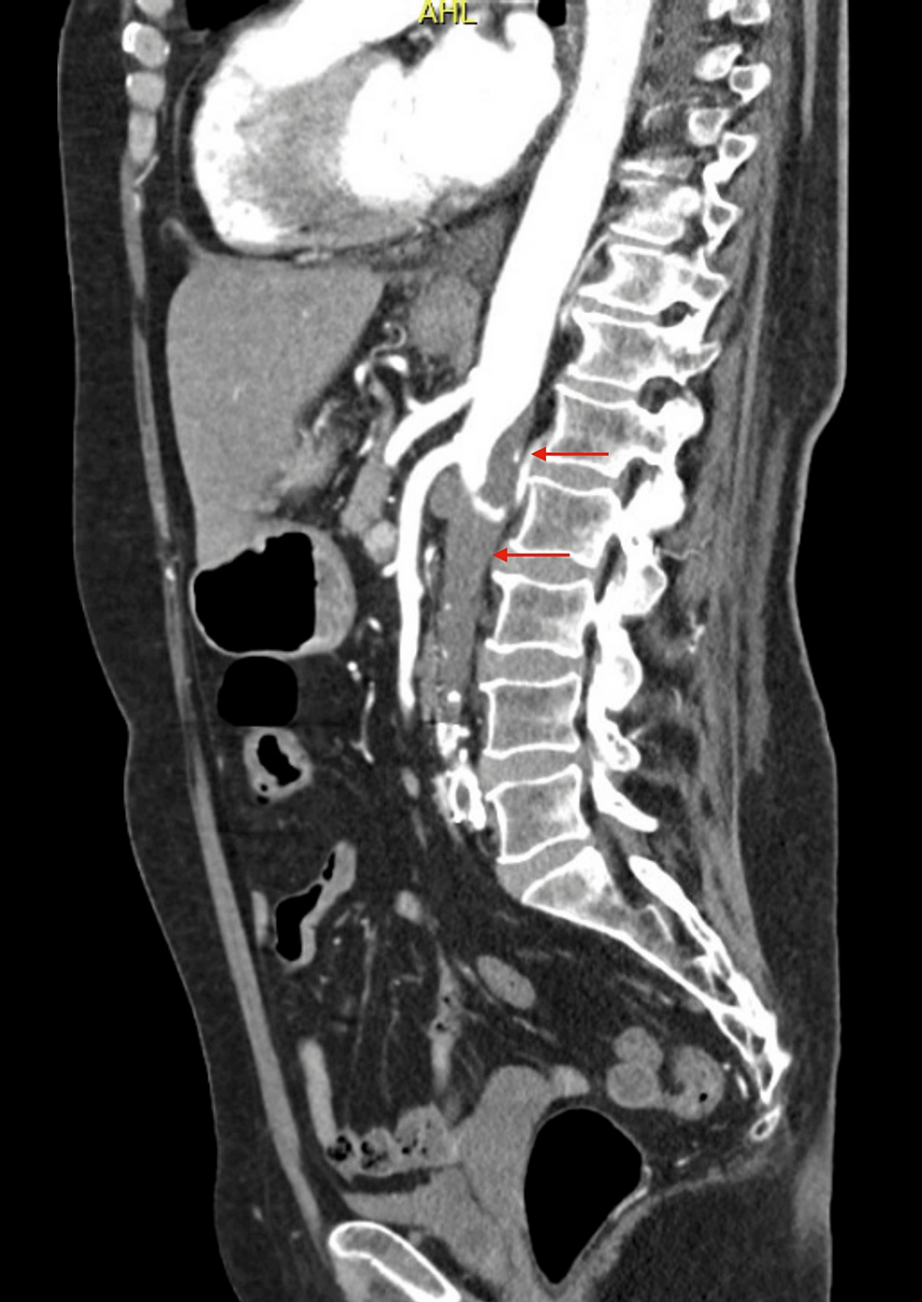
Abdominal CT angiography showing an almost complete opacification defect of the abdominal aorta (red arrows), below renal branches, with features suggesting chronic stent thrombosis.
